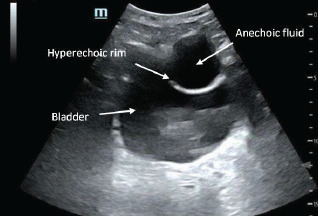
Point-of -care ultrasound image of anechoic fluid collection with hyperechoic rim lateral to bladder in transverse view.
